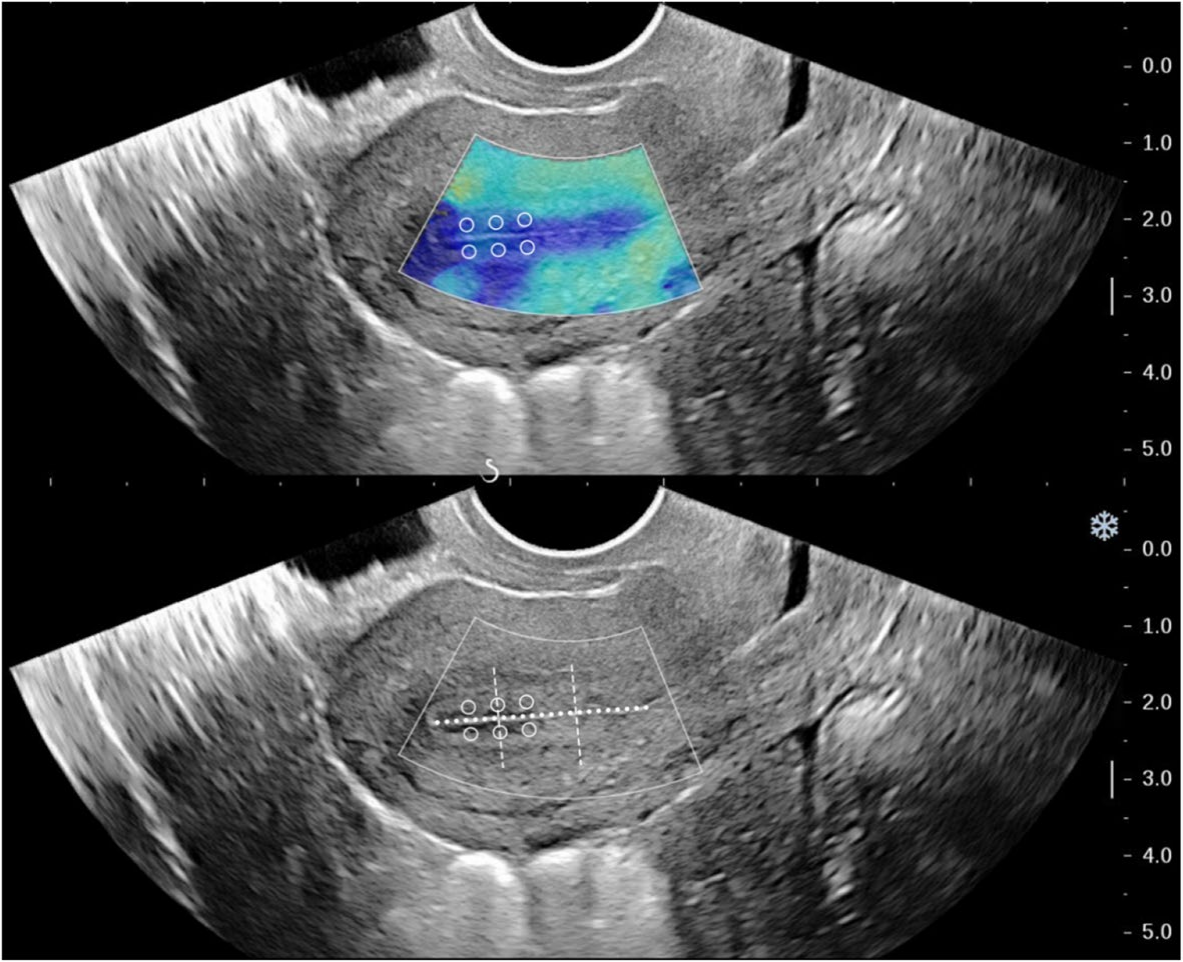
Example sonographic image of the region of interest (ROI) used for measuring endometrial elasticity using SWE. Notes: In a standard sagittal section of the uterus, the endometrium is completely covered by the white sampling frame; the white horizontal dotted line along the uterine cavity line is of the same length as the endometrium; the other two white dotted lines are vertical to the endometrial line and divide the endometrium into three segments; the white circles indicate the six sampling sites for SWE located in the middle and upper segments of the endometrium
